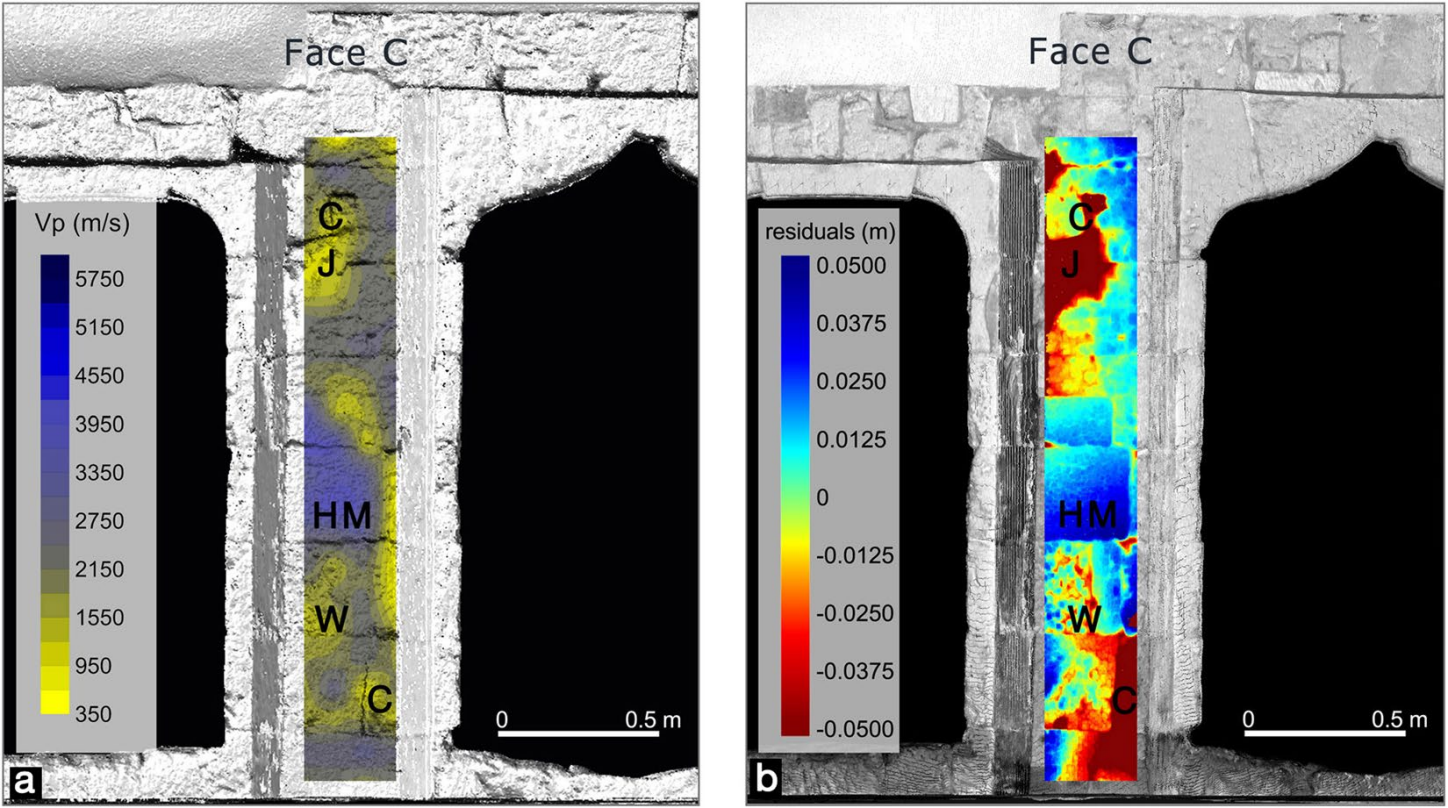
Comparison between the ultrasonic map and the TLS results on face C of the pillar. The two techniques of the NDT survey show matching results highlihted by uppercase letters. (C = crack; J = Junction; W = Weathering; HM = Health Material).
